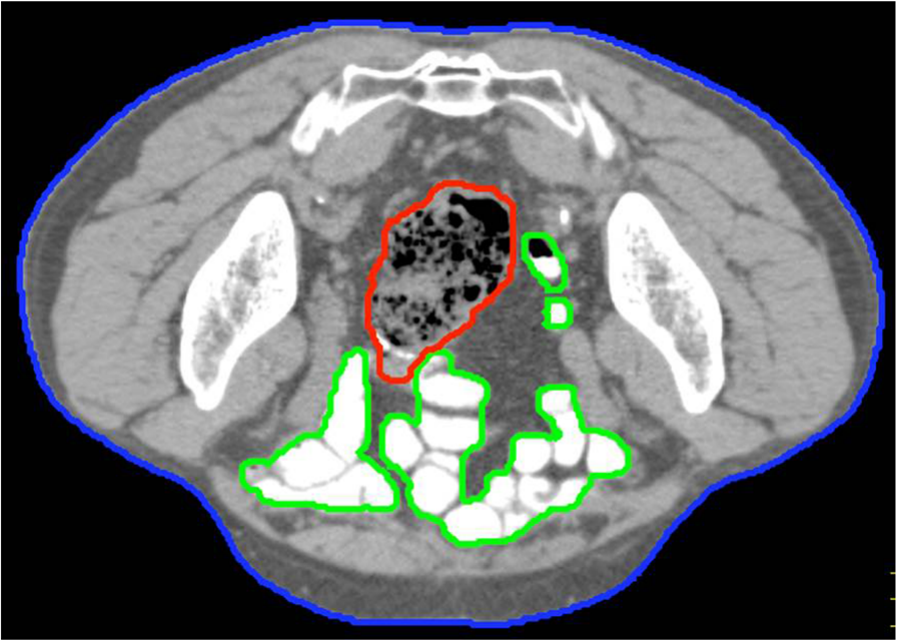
A representative CT image captured after the administration of gastrografin *Blue* skin, *green* small intestine, *red* sigmoid colon
